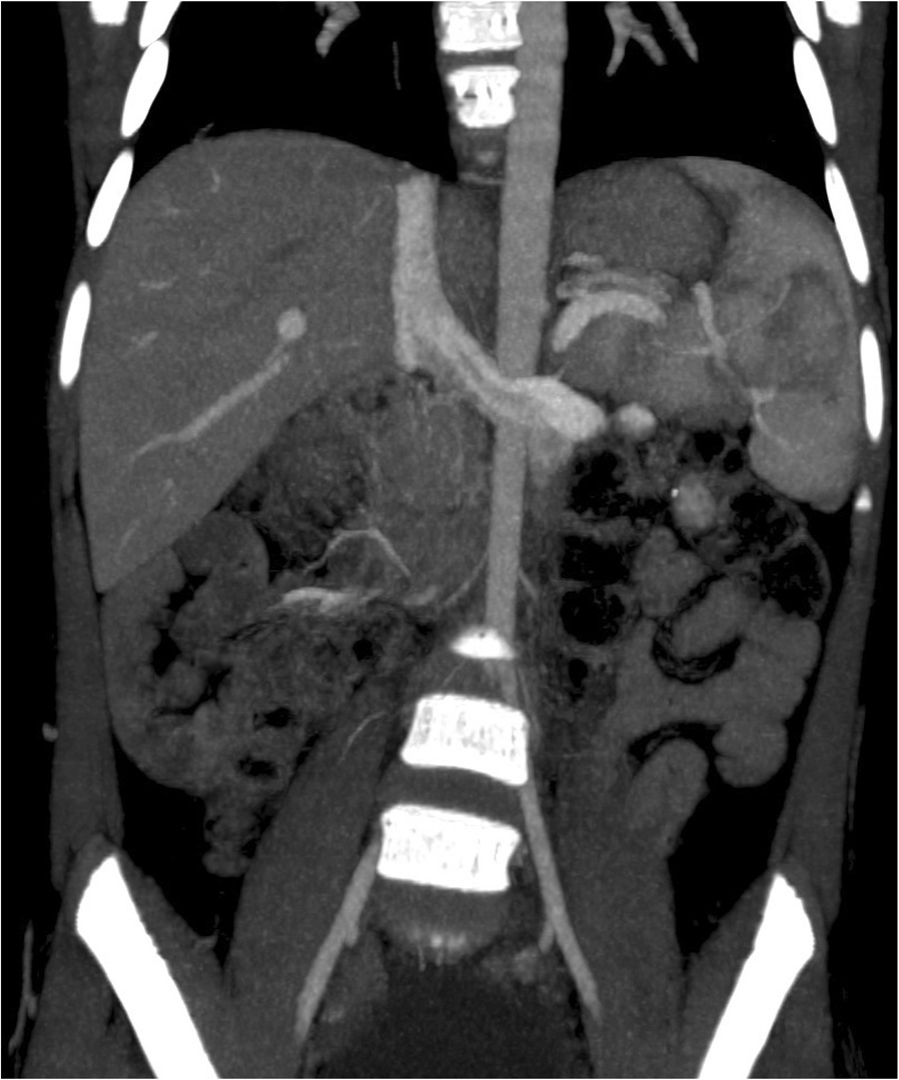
Computed tomography venogram (coronal section) showing normal drainage of renal vein into inferior vena cava with complete absence of infrarenal inferior vena cava
